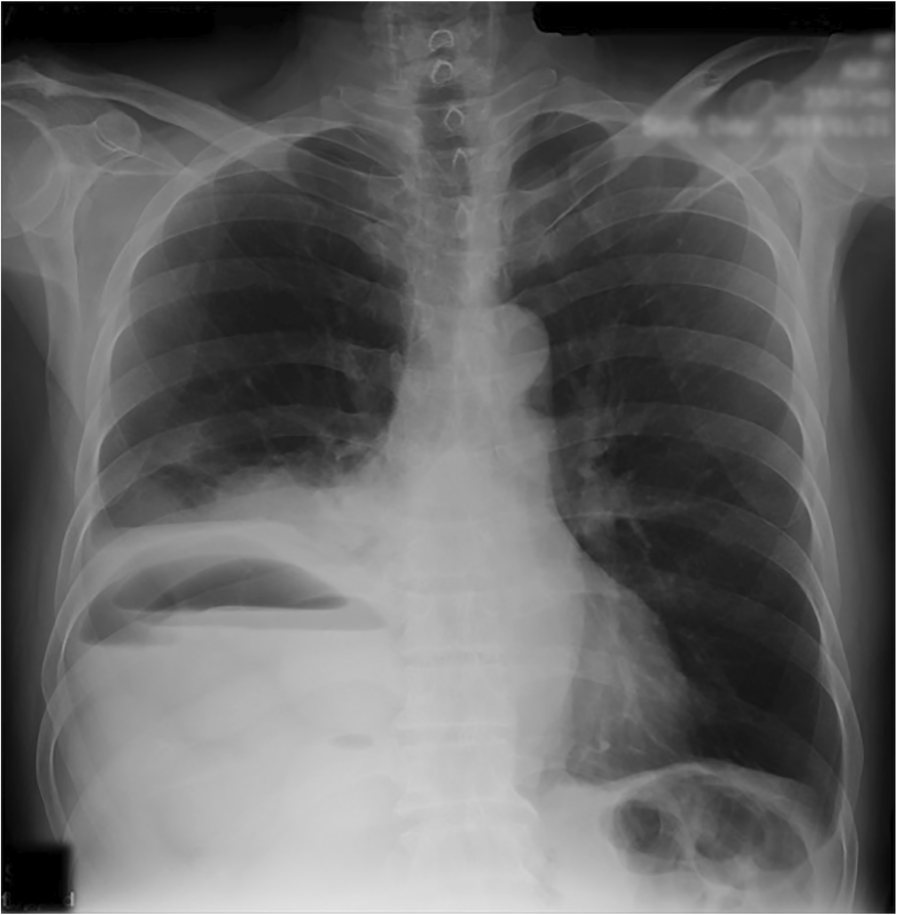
Chest X-ray: air accumulation above the liver
